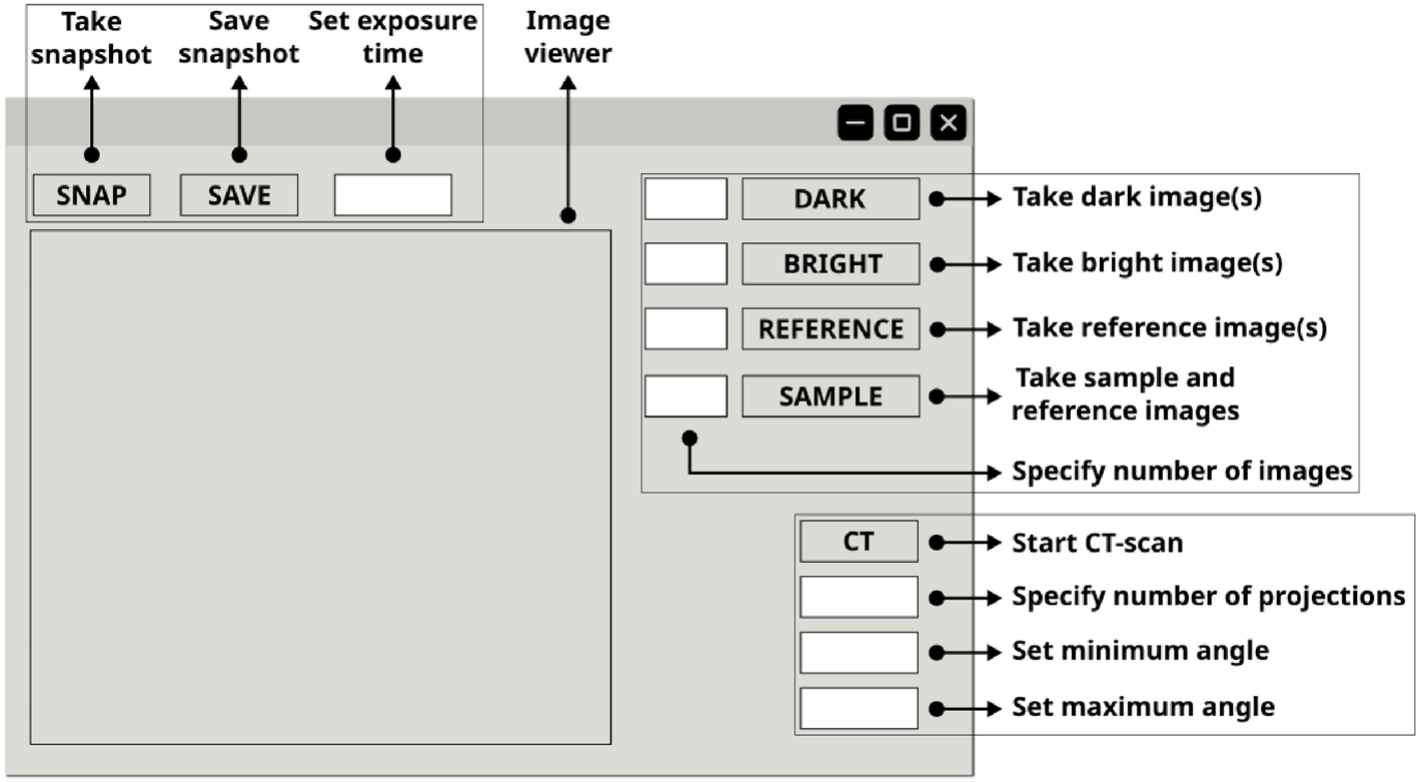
Graphical user interface (GUI) designed for the image acquisition procedure. The GUI is straightforward and user-friendly, featuring two primary operation modes: single projection imaging and computed tomography (CT). Acquisition of dark, bright, and reference images is common to both modes. Additionally, the interface includes real-time visualization of acquired images, camera controls for adjusting exposure time, and snapshot functionality, allowing temporary image inspection without affecting the organized measurement directories.
